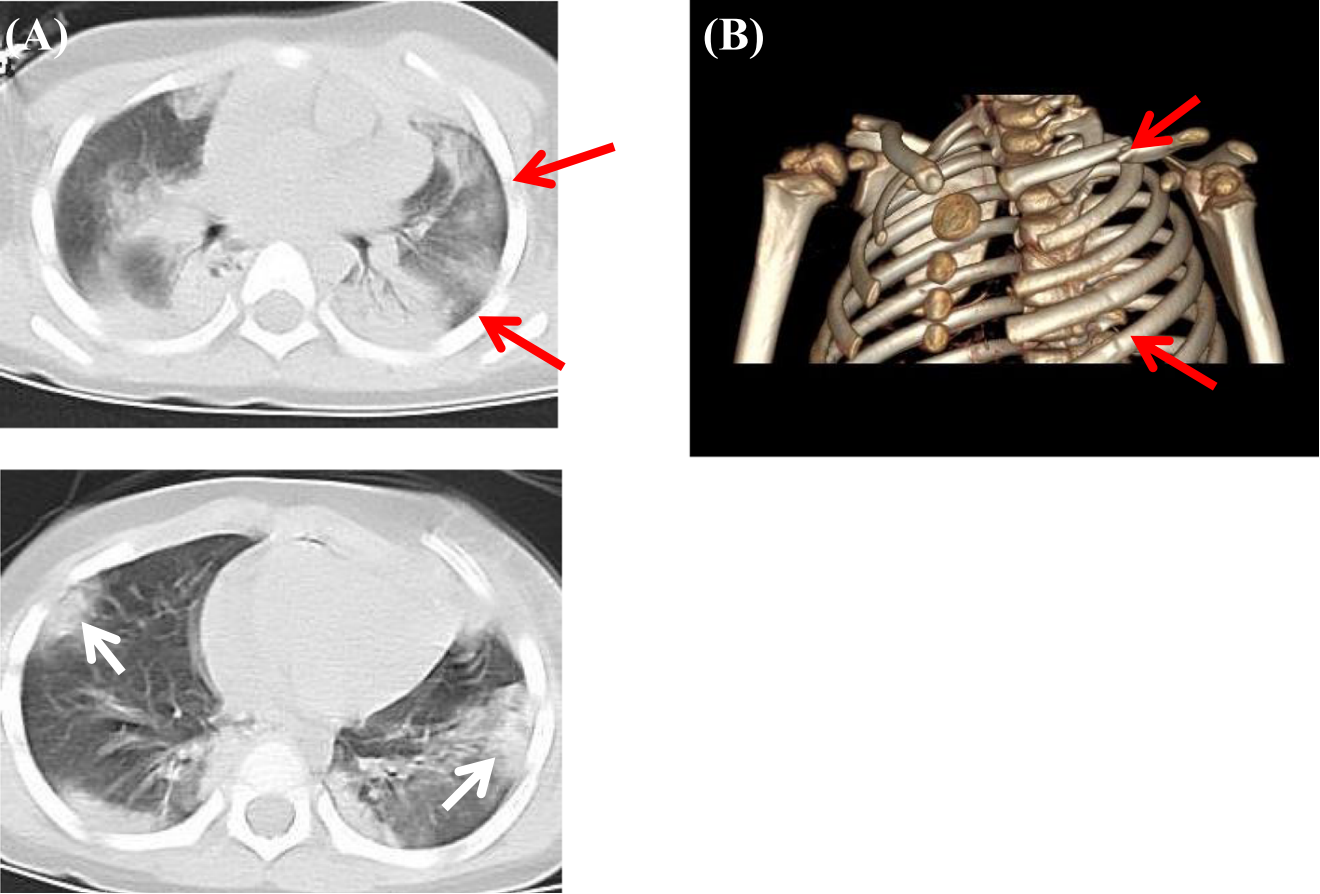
Chest CT of Case 1 on Admission. a Two-dimensional axial CT scan showed lung contusions (*white arrows*) and left pneumothorax (*red arrows*). b Three-dimensional reconstruction of the CT scan showed fractures of the left clavicle and the third to fifth ribs on the left (*red arrows*)
